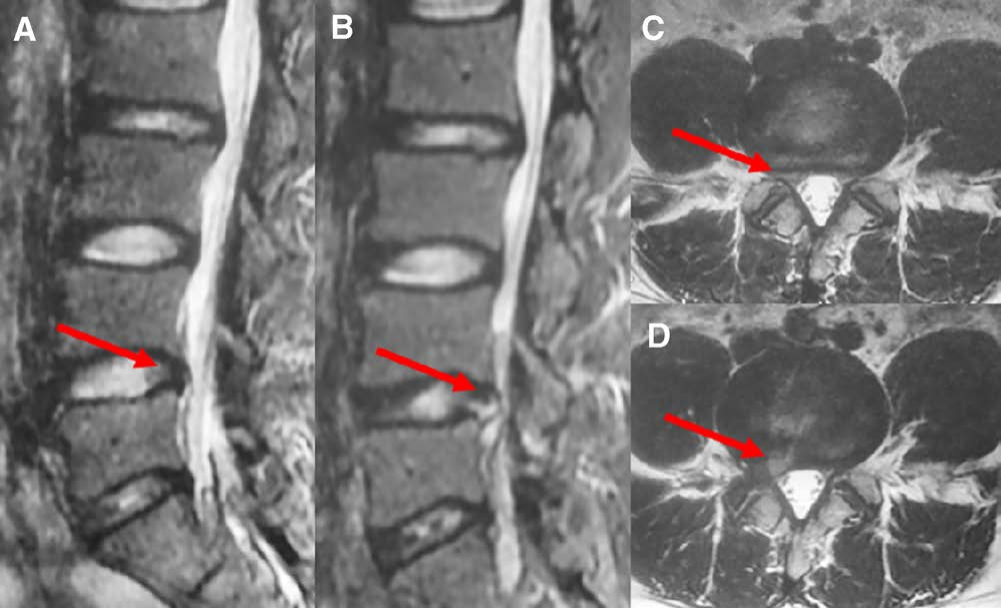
MRI images of Case 2 at the first and second admissions. (A, C) MRI of case 2 on the first admission showed that the L4/5 intervertebral disc herniated posteriorly to the right, compressing the dural sac. (A) T2-weighted sagittal image. (C) T2-weighted cross-sectional image. (B, D) MRI of case 2 on the second admission showed an enhanced signal in T2-weighted images at the L4/5 level. (B) T2-weighted sagittal image. (D) T2-weighted cross-sectional image.
